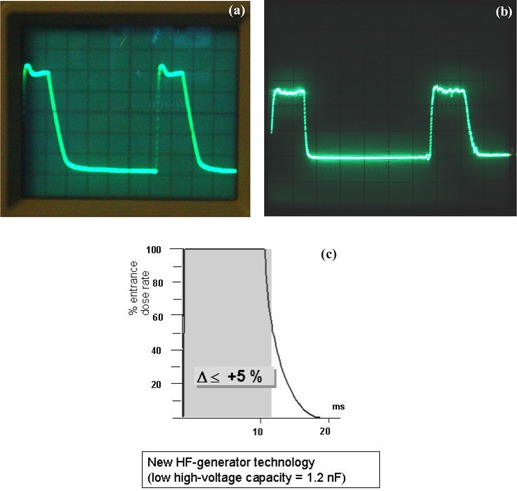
(a). A display of the tube potential waveform measured directly from the X‐ray tube using a Dynalyzer III system (Machlett Laboratories, CT) and an oscilloscope display. The oscilloscope was set at 10 ms/scale horizontally and 20 kV/ scale vertically. The technique of the X‐ray system was 98 kVp, 3.8 mA, 15 pps, and 0.2 mm Cu filter. (b) A display of the exposure rate waveform measured at the entrance of a block of 1.5” type 1100 aluminum, 2.0 mm copper, and 3.0 mm lead using an MDH ion chamber system and an oscilloscope display (set at 10 ms/scale horizontally). The technique was 110 kVp, 4.1 mA, 15 pps, and 0.2 mm Cu filter. The exposure rate was read 4.1 R/min. (c) The percentage of exposure rate at the skin entrance during one pulse in pulsed fluoroscopy mode is compared with a sharp cutoff square pulse by a grid‐controlled X‐ray tube. This was obtained with 12‐m cable length, 67 kV tube voltage, and 0.2 mm Cu filter (courtesy of Siemens Medical Solutions).
